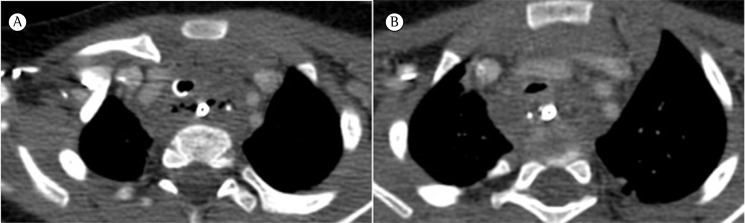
CT scans showing upper mediastinum widening with areas of low attenuation
